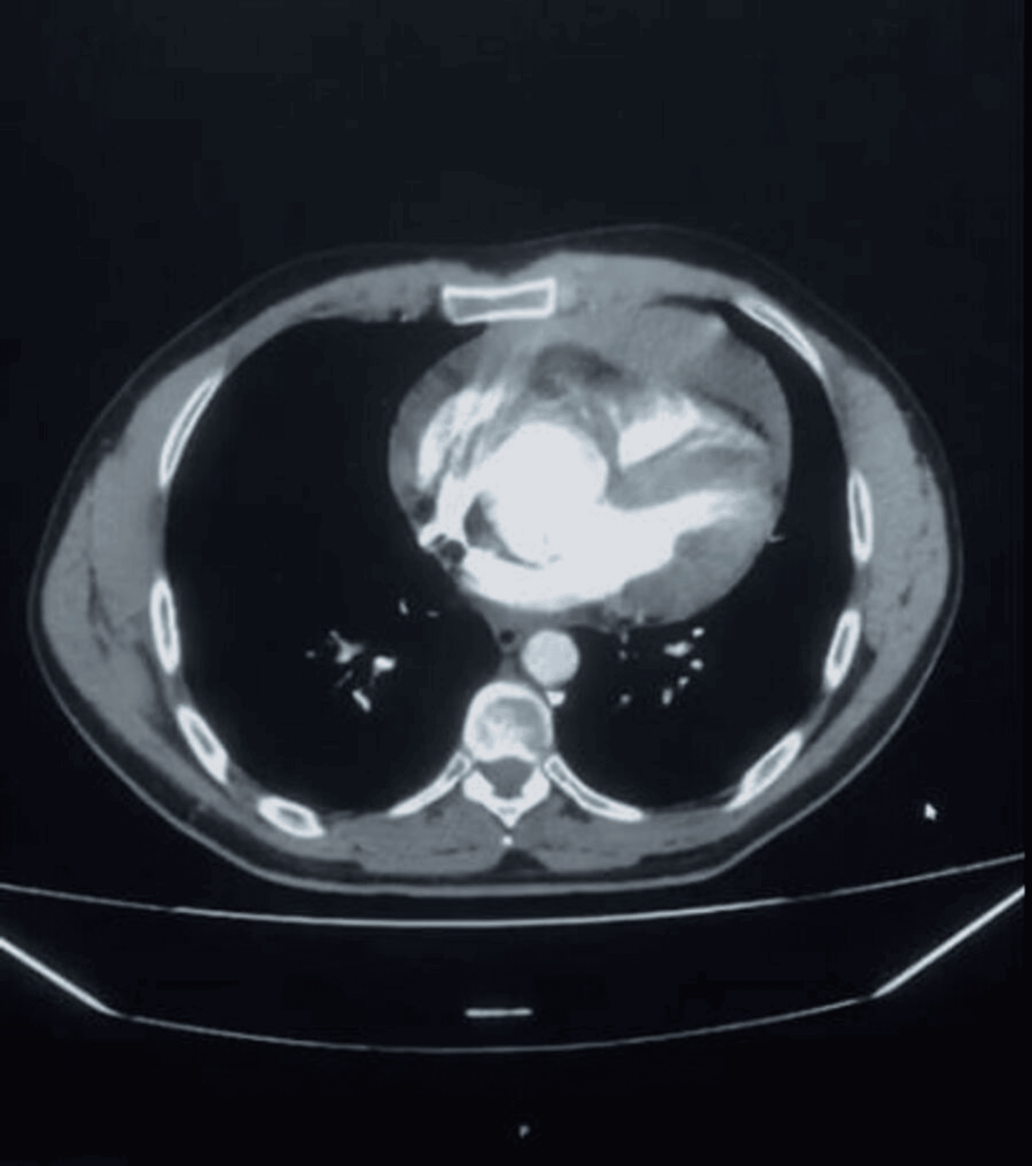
CT with contrast showing the aortic dissection with dilation of the root.
